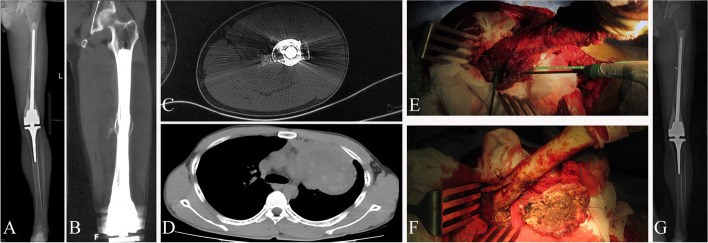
A 23-year-old male patient with ﻿with recurrent osteosarcoma in the middle femur treated by tumor microwave ablation and resection. A, B, C X-ray and CT show recurrent osteosarcoma in the middle femur at 3 years of after tumor bone inactivation combined with prosthesis reconstruction. D CT shows lung metastasis. E Recurrent osteosarcoma accepted microwave ablation before resection. F After microwave ablation, the degenerative tumor tissue accepted resection only. G Re operation without other treatments does not affect the stability of the prosthesis
